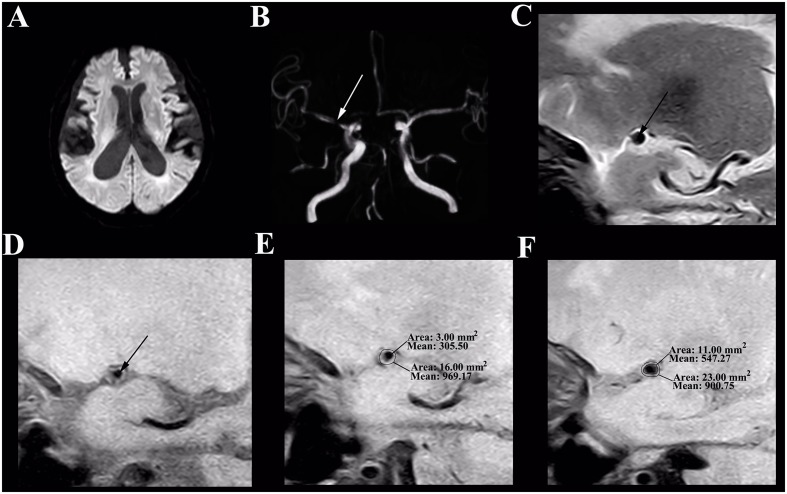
HR-MRI of an asymptomatic MCA stenosis in an 82-year-old male who presented with aphasia for 1 day. (A) DWI shows normal. (B) The TOF-MRA manifests severe stenosis of the M1 segment of right MCA (white arrow). (C) The plaque in T2-weighted HR-MRI (black arrow). (D) The plaque in T1-weighted HR-MRI (black arrow). (E) Measurement at the most narrowed site in proton density-weighted HR-MRI: the vessel area is 16.00 mm^2^ and the lumen area is 3.00 mm^2^. (F) Measurement at the reference site in proton density-weighted HR-MRI: the vessel area is 23.00 mm^2^ and the lumen area is 11.00 mm^2^. The remodeling index = 16.00 mm^2^/23.00 mm^2^= 0.70 (negative remodeling); the degree of stenosis = (1–3.00 mm^2^/11.00 mm^2^) = 72.73%.
